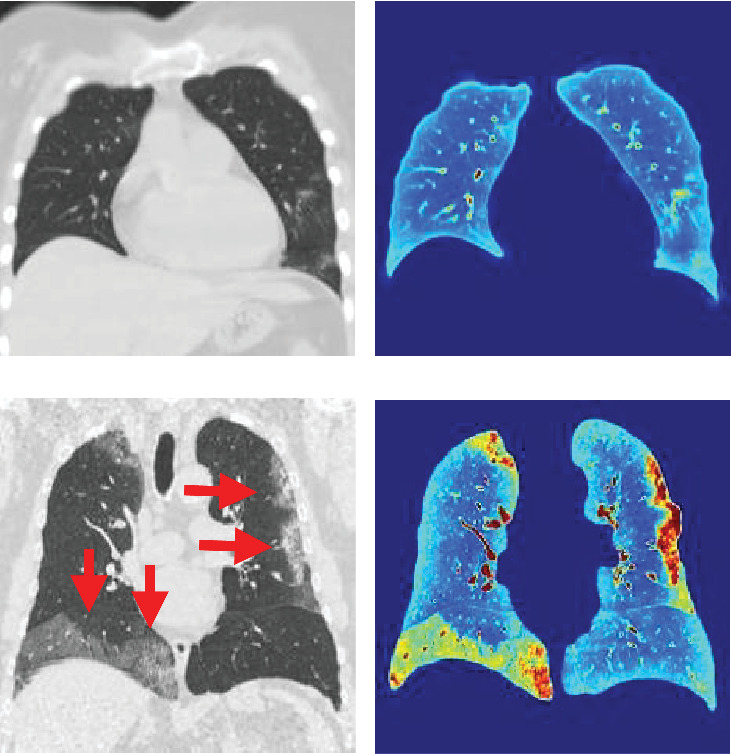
Computed tomography of the chest of a patient with nonserious COVID-19. (a) Without processing. (b) With processing. CT scan of a severe COVID-19 patient. (c) Without processing. (d) With processing.
